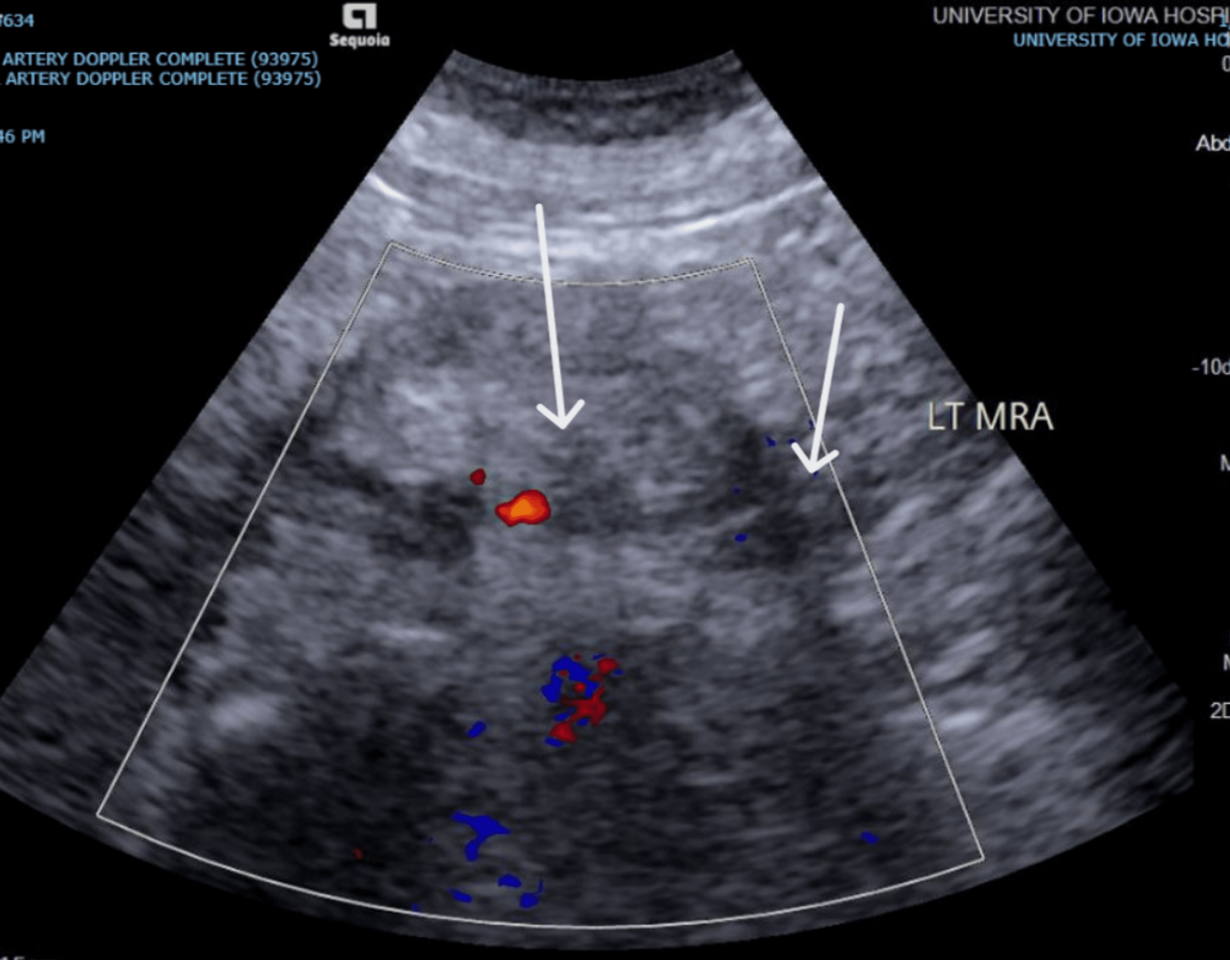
Doppler ultrasound of the renal arteries. Absent color flow signals within the left renal artery (arrows).
